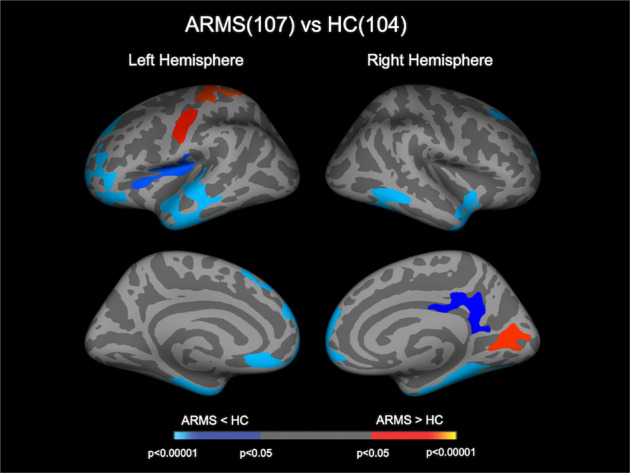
Clusters showing differences in CT between ARMS individuals and HC. Cortical statistical maps displaying altered CT in individuals with ARMS compared with HC. The maps are shown for the right and left hemispheres in lateral and medial views, respectively. The horizontal bar shows *p* values corrected for multiple comparisons. ARMS at-risk mental state, CT cortical thickness, HC healthy control.
